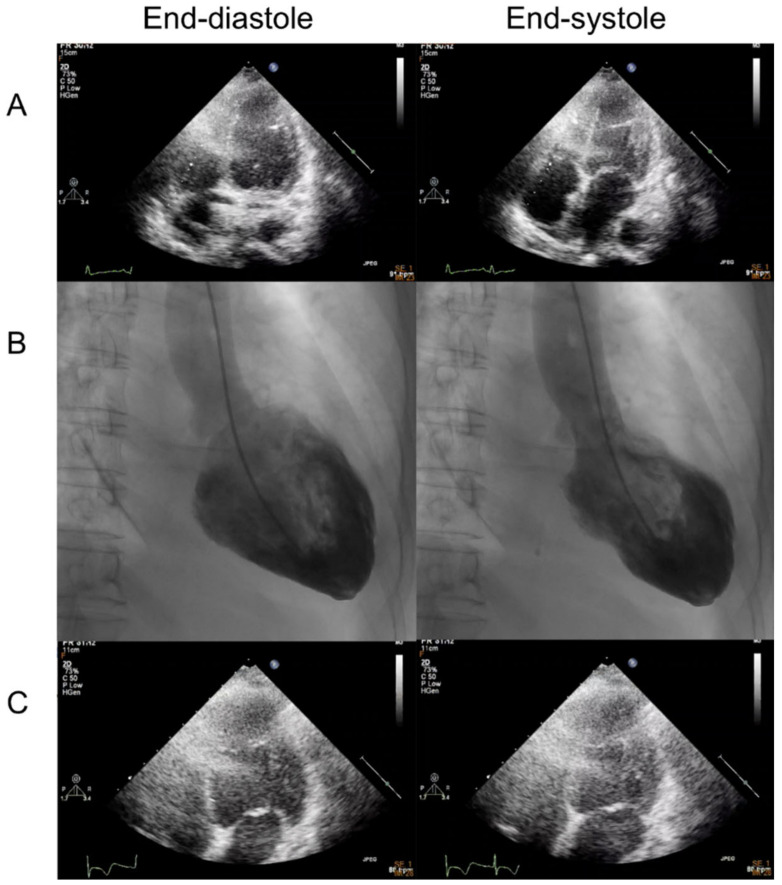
Left ventriculogram and serial echocardiogram of Takotsubo syndrome occurring after COVID-19 mRNA vaccination. (A,B) Echocardiography (four-chamber images) and left ventriculography at the presentation of Takotsubo syndrome after COVID-19 mRNA vaccination. (C) Repeat echocardiography (four-chamber images) revealed an improvement in apical ballooning on day 6. Notice the slow recovery of the negative T wave during the subacute phase (left panel: end-diastolic phase, right panel: end-systolic phase).
